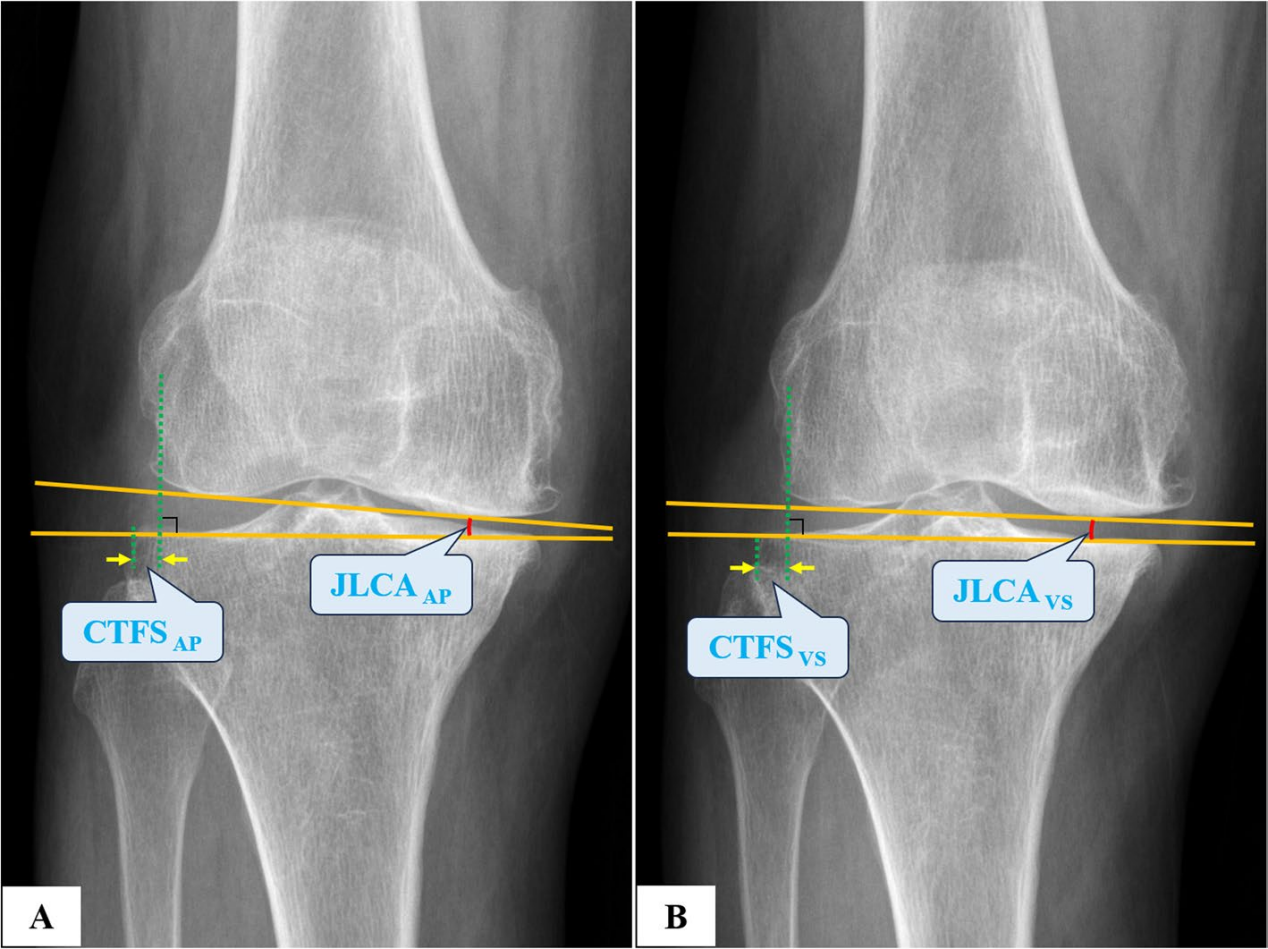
Measurement of parameters on the non-weight-bearing AP knee radiographs. Using the same method shown in Fig. 1C, CTFS and JLCA were measured on regular AP (A) and VS radiographs (B)
